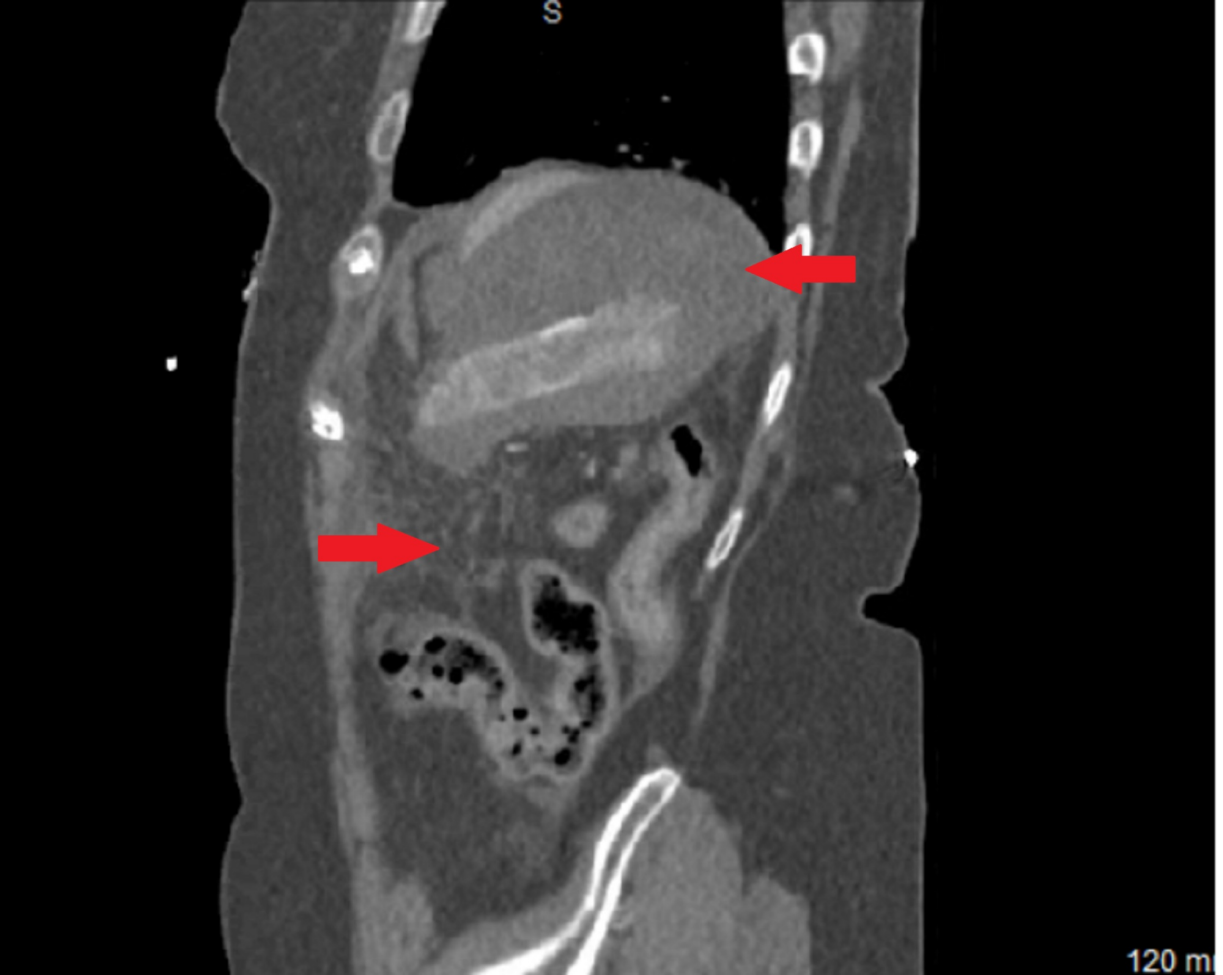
Contrast-enhanced abdominal computed tomography (sagittal view) with red arrows showing perisplenic and peritoneal fluid
